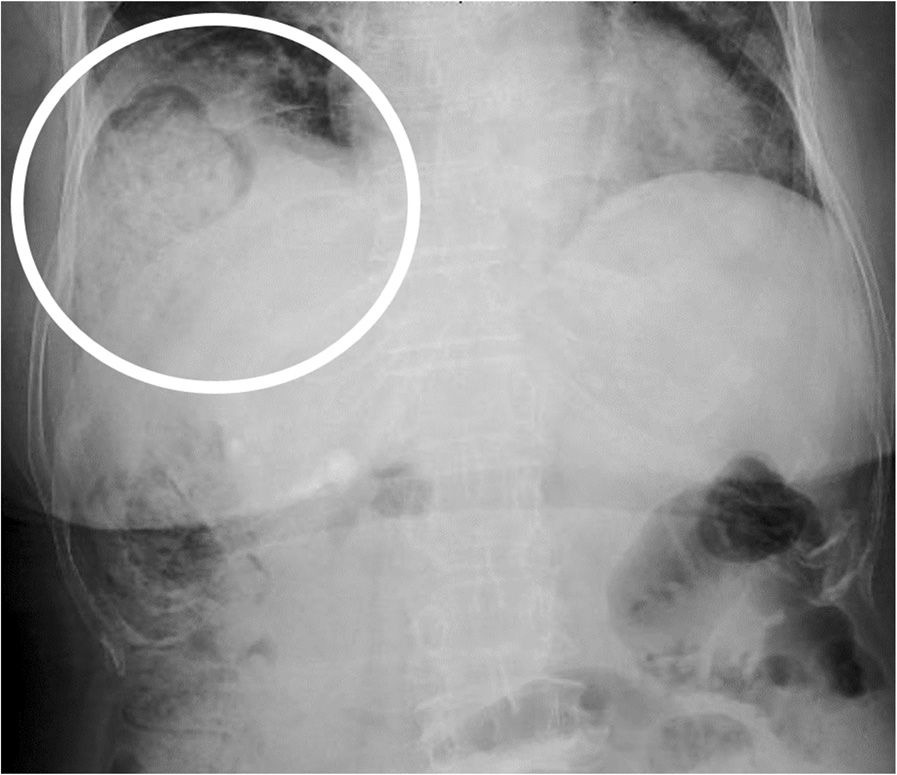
Abdominal radiograph obtained at symptom onset, showing the deviation of the colon across the right diaphragm (*white circle*)
